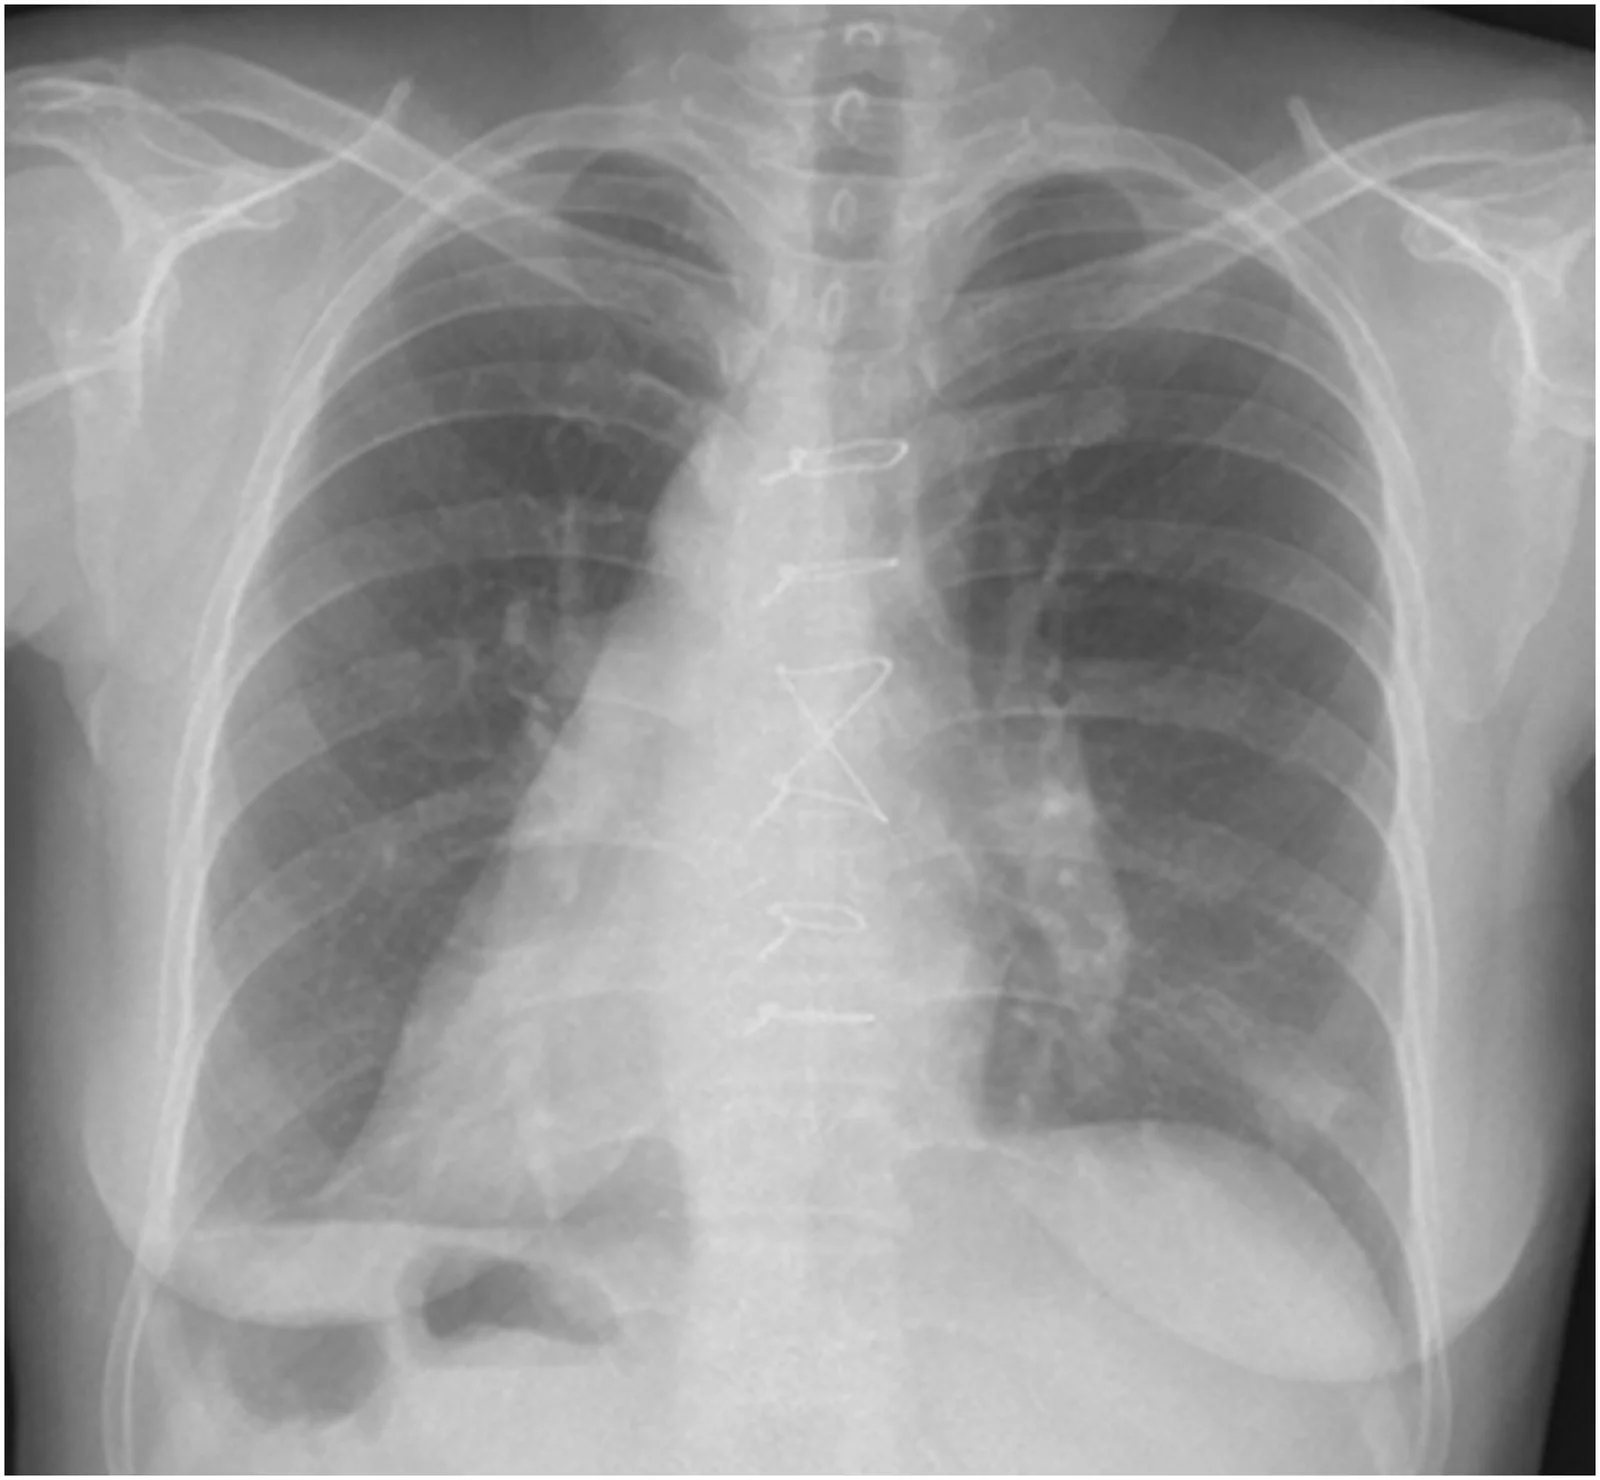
Chest X-ray. Post-sternotomy changes are noted. Dextrocardia of the heart is present.
